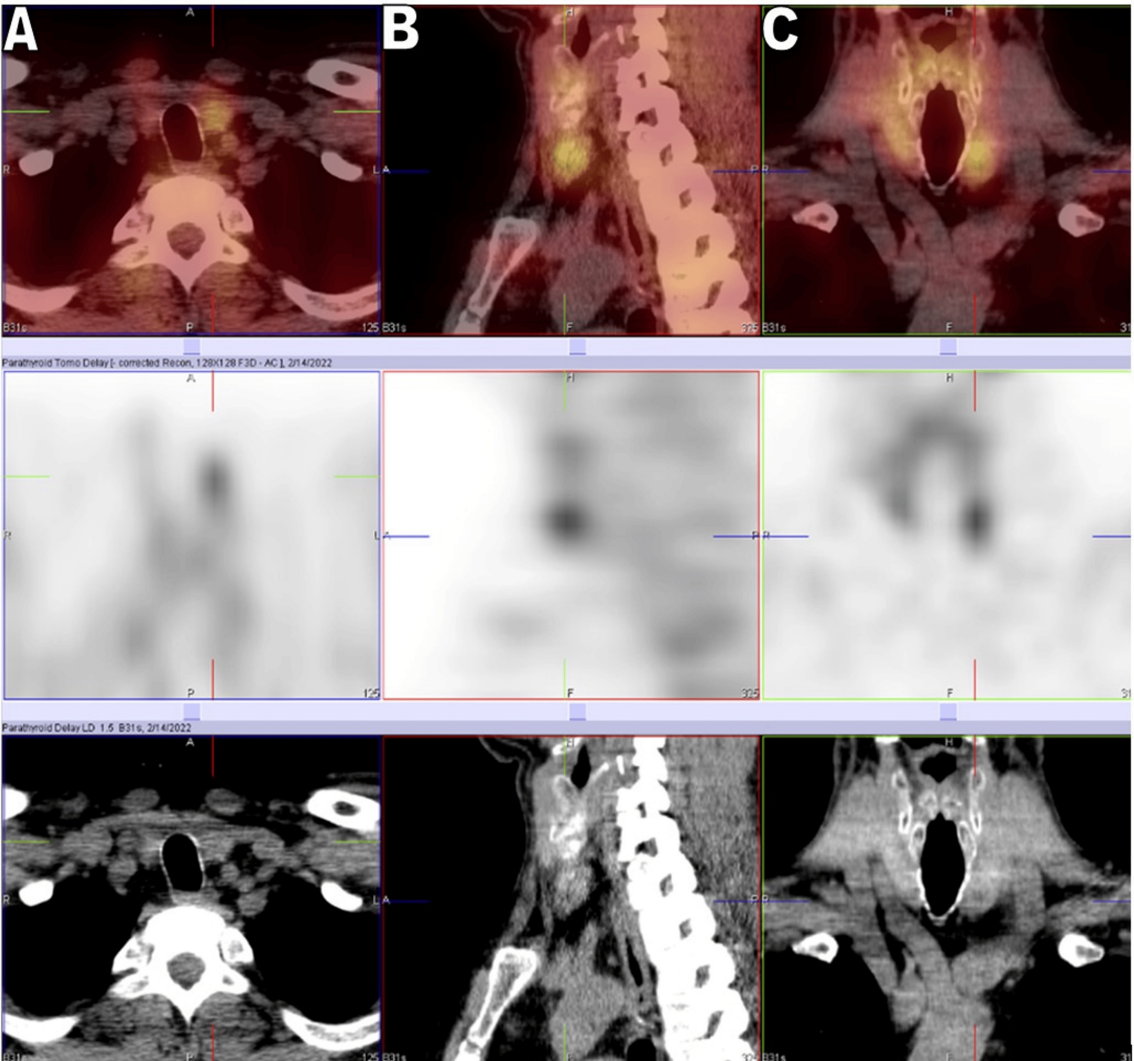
The SPECT/CT study following IV administration of 21 mCi Tc-99m labeled sestamibi. Images were acquired at 30 minutes and 2.5 hours. There is a small focal uptake at the inferior pole of the left lobe of the thyroid gland, which persists in the delayed phase, suspected to be a parathyroid adenoma. (A) Transverse view; (B) Sagittal view; (C) Coronal view SPECT: Single photon emission computed tomography
